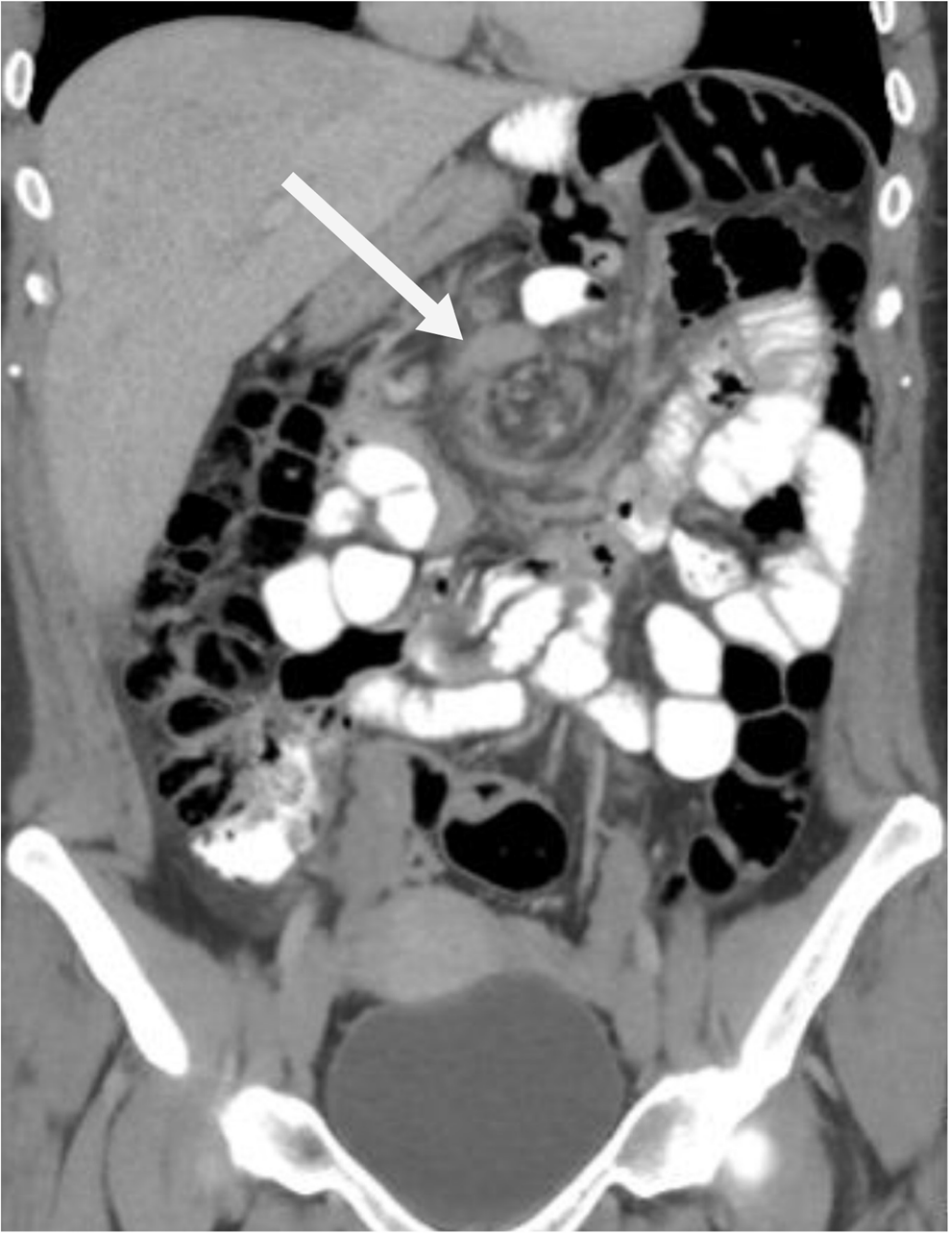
Sagittal CT scan of a 360° swirl sign in the mesenteric vessels of a 34-year-old woman with internal herniation
